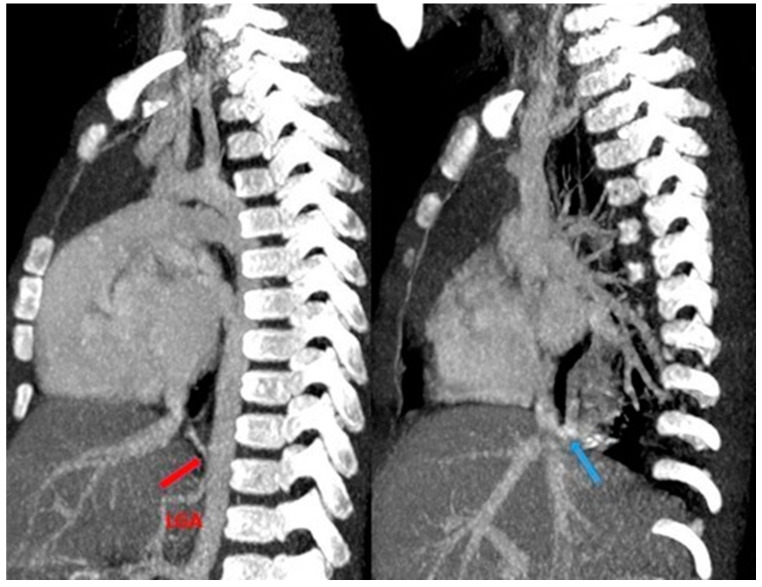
CT angiography with maximum intensity projection (MIP) reconstruction shows an anomalous artery (red arrow) arising from left gastric artery (LGA) into sequestered lung and an anomalous vein (blue arrow) draining into inferior vena cava, in its intrahepatic tract. These findings are compatible with extra-lobar sequestration.
